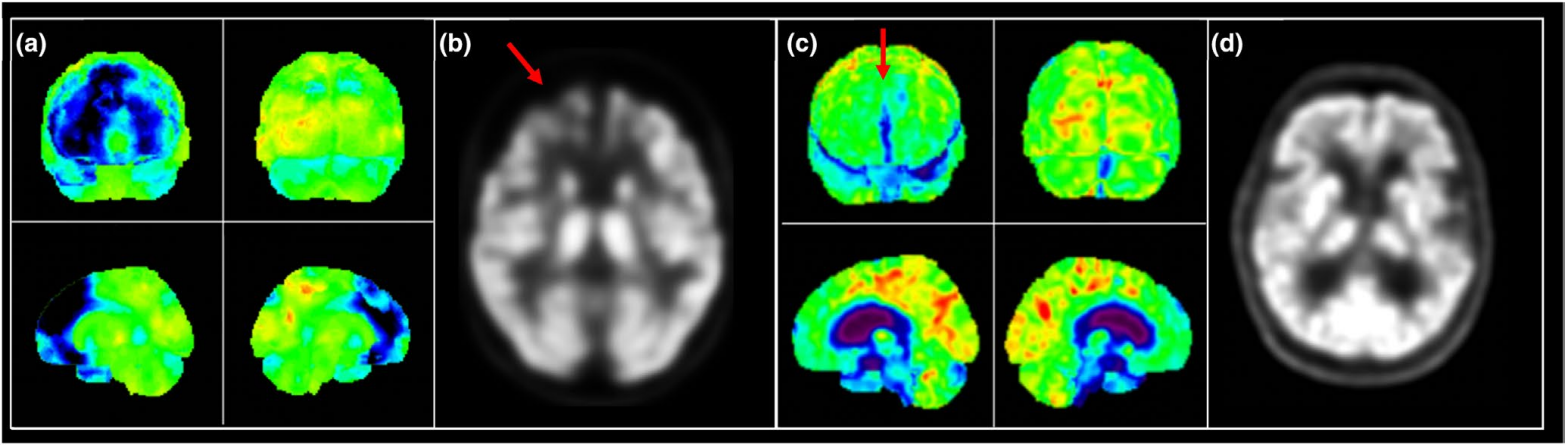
FDG‐PET scans of a true‐ and false‐positive patient both reported to have frontal lobe hypometabolism suggestive of bvFTD. (a) Stereotactic surface projection of a true‐positive bvFTD patient showing obvious frontal lobe hypometabolism and their grey‐scale image also showing obvious frontal lobe hypometabolism (b, arrow). (c) Stereotactic surface projection of a false‐positive patient who did not meet criteria for a clinical disorder. A registration artefact within the interhemispheric fissure was misinterpreted as hypometabolism (arrow) while their grey‐scale image showed normal glucose metabolism (d).
